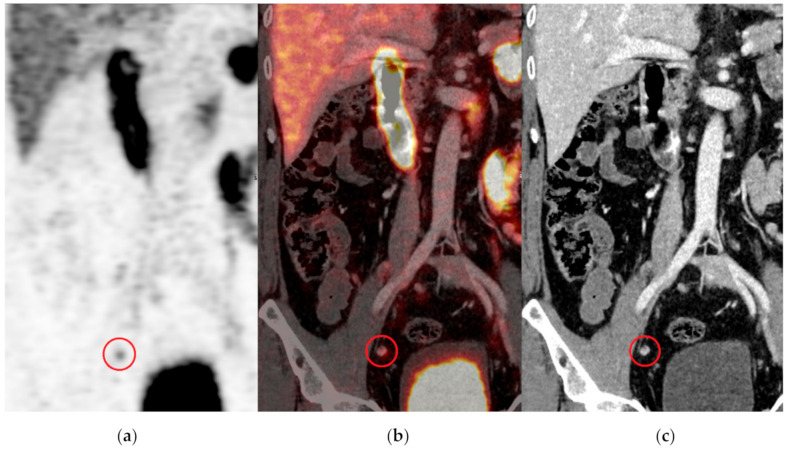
Coronal projections of (a) a PET, (b) PET-CT fusion and (c) CT using the OSEM reconstruction algorithm. An equivocal lymph node can be seen in the pelvis (encircled). Note the similar signal intensity of the lymph node compared to the liver uptake.
